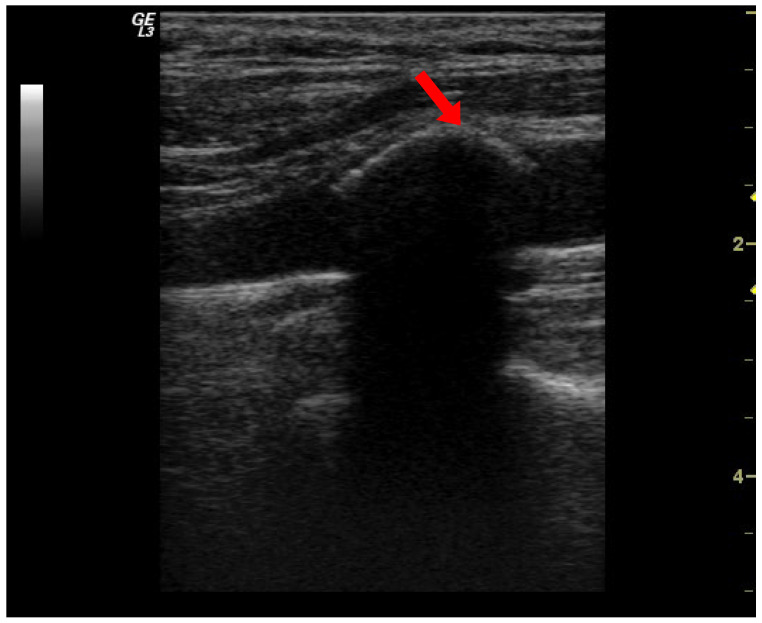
Ultrasound examination. Common carotid artery calcification is the patchy hyperechoic image with a posterior shadow in the arterial wall (red arrow).
